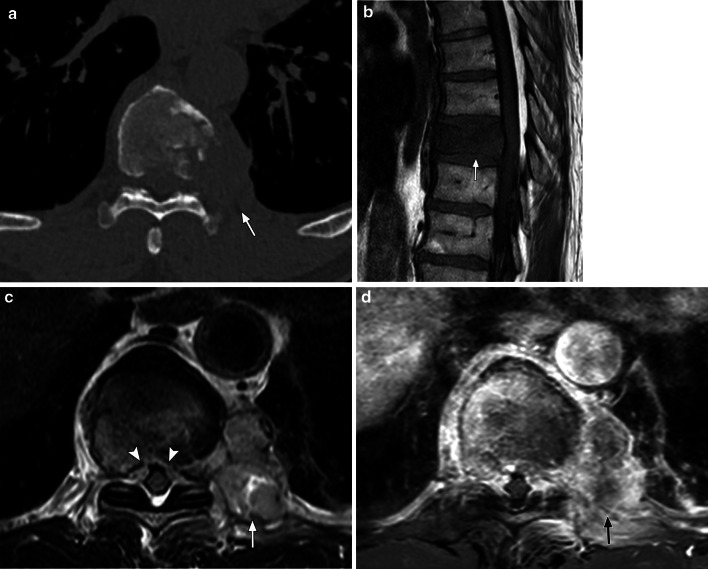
61-year-old man with primary bone angiosarcoma. a Axial CT (bone windows) image shows a destructive lesion centred at the left T9 vertebral body with extra-osseous soft tissue (arrow). b Sagittal T1-weighted image shows low signal change of the T9 vertebral body (arrow) and bulging of the posterior cortex in keeping with malignant infiltration. c Axial T2-weighted image shows infiltration of the T9 vertebral body with a heterogeneous, predominantly high signal intensity left paravertebral mass (arrow) involving the adjacent rib and pleura. There is extra-osseous soft tissue narrowing the spinal canal (arrowheads). d Axial T1 fat-suppressed post-contrast image shows avid heterogeneous enhancement of the T9 vertebral body and the left paravertebral mass (arrow)
